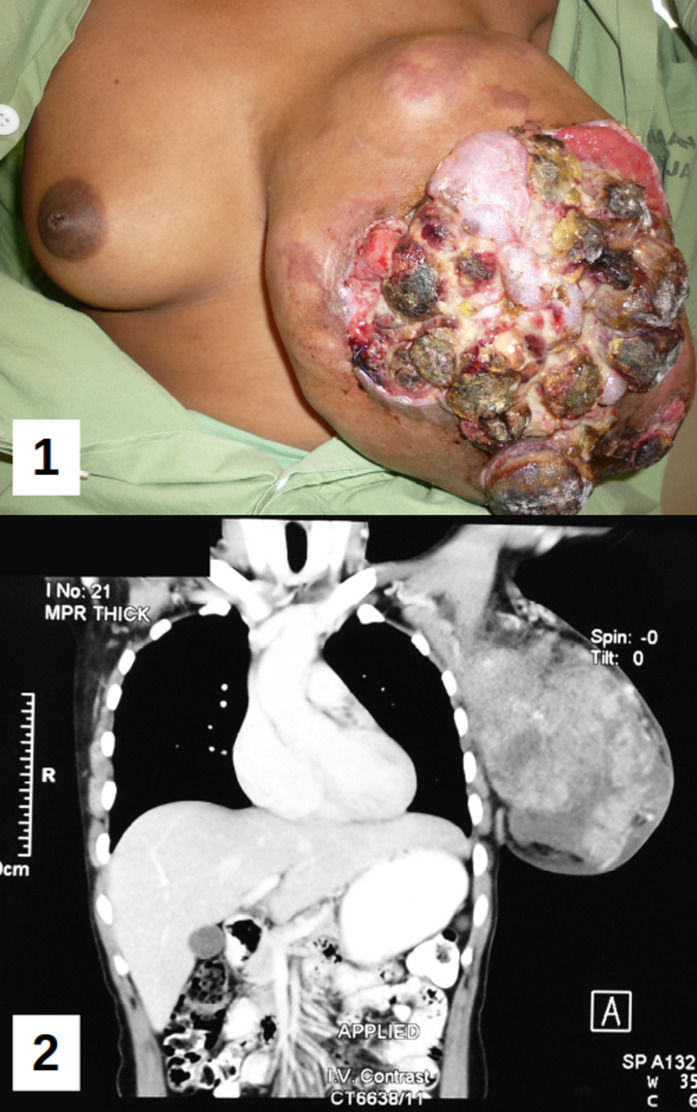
A) ulcerative cauliflower like Lump of the left breast; B) computed tomography (CT) image of the left breast showing hypo and hyperdense areas
